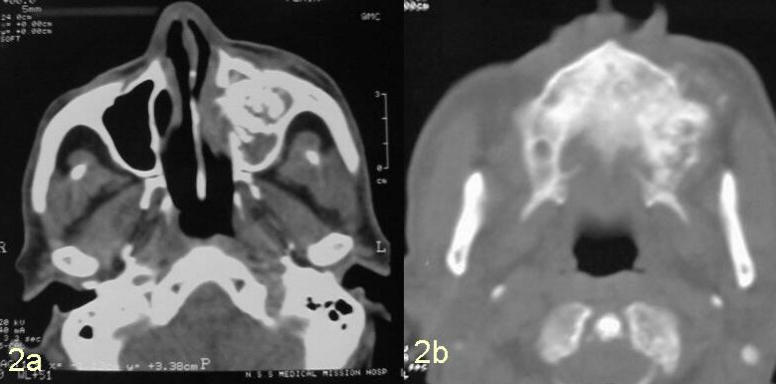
Computed tomographic scan showing lesion in maxillary antrum with destruction of maxilla (2a) and hard palate (2b).
